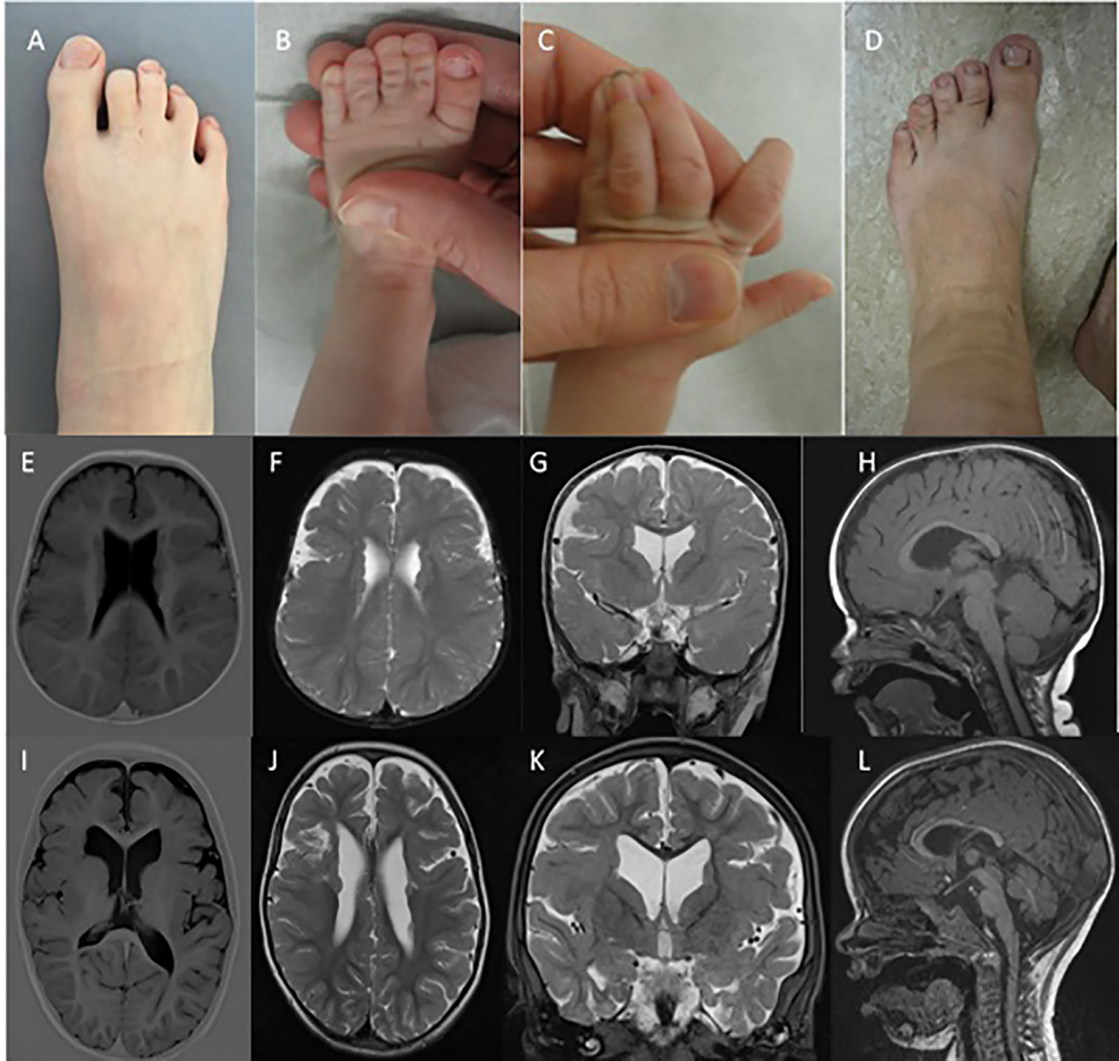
Syndactyly phenotypes and brain MRI. (A D) indicate the presence of variable syndactyly in case 1 (A), case 2 (B, C), and the mother of case 2 (D). Brain MRIs of case 1 (E–H) and case 2 (I–L). Mild ventricular dilatation, bilateral PNH, and bilateral PMG are demonstrated in both cases (E-G, I-K). (H, L) demonstrate thinning of the corpus callosum with normal infratentorial findings.
